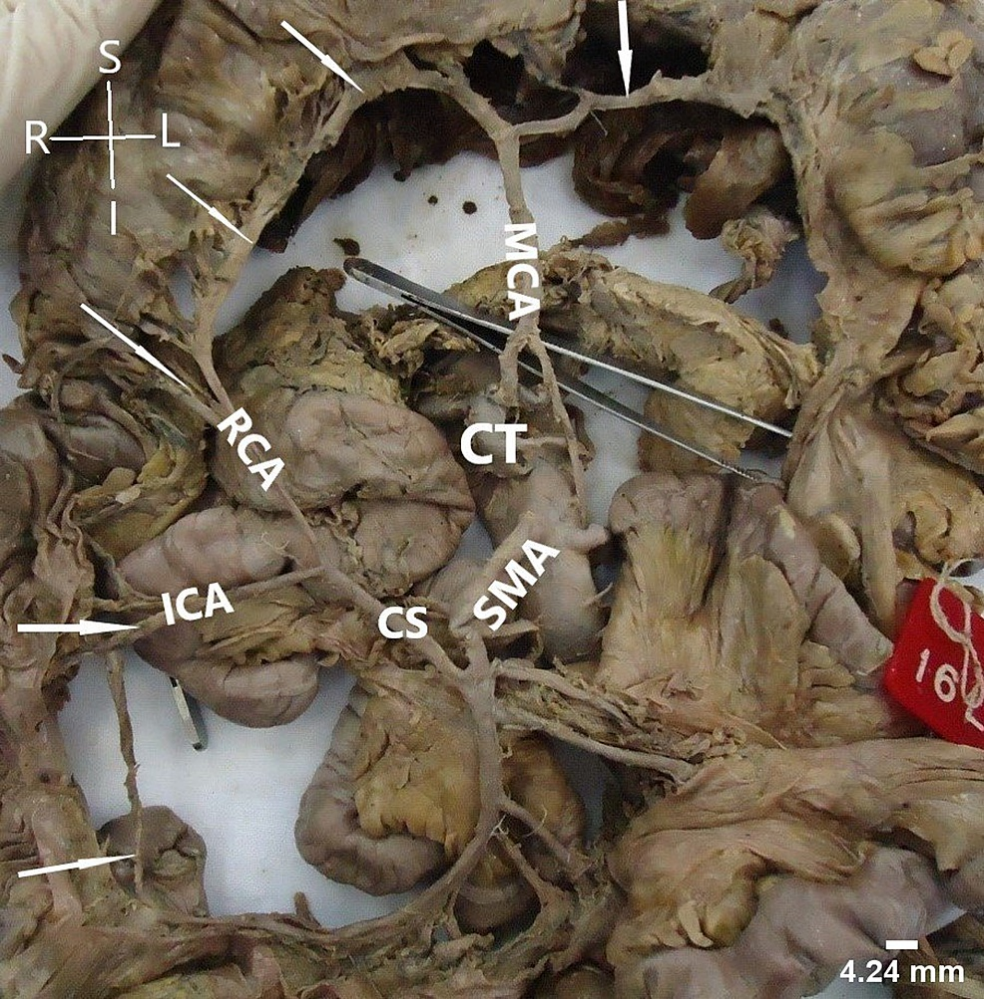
Common stem (CS) of origin of right colic artery (RCA) and ileocolic artery (ICA) from superior mesenteric artery (SMA). The image shows the middle colic artery (MCA) arising from celiac trunk (CT). The arrows show the formation of inter-colic anastomotic artery.
